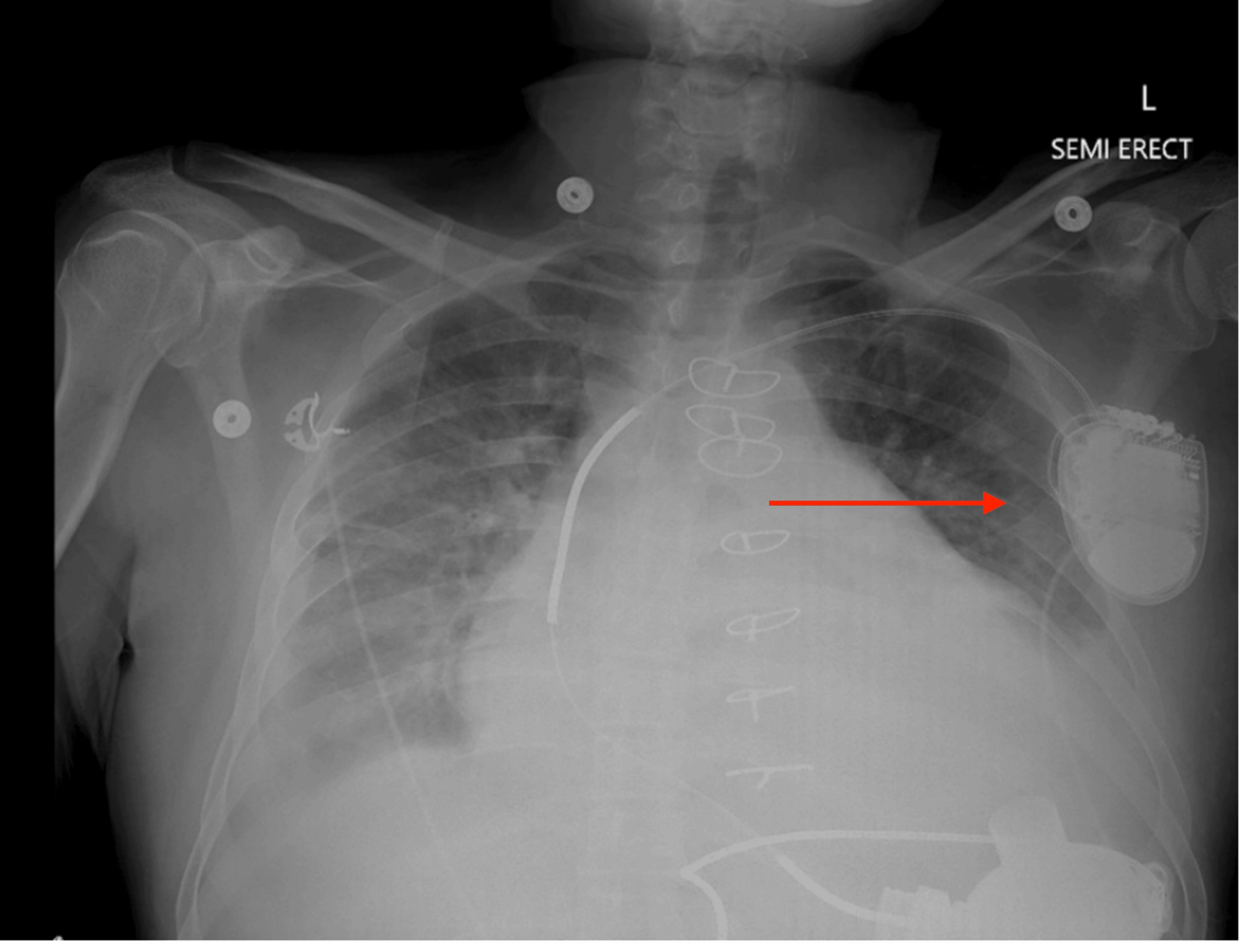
Chest X-ray demonstrating stable postoperative changes of median sternotomy and a left ventricular assist device in place (arrow), along with an automatic implantable cardioverter-defibrillator on the left side
